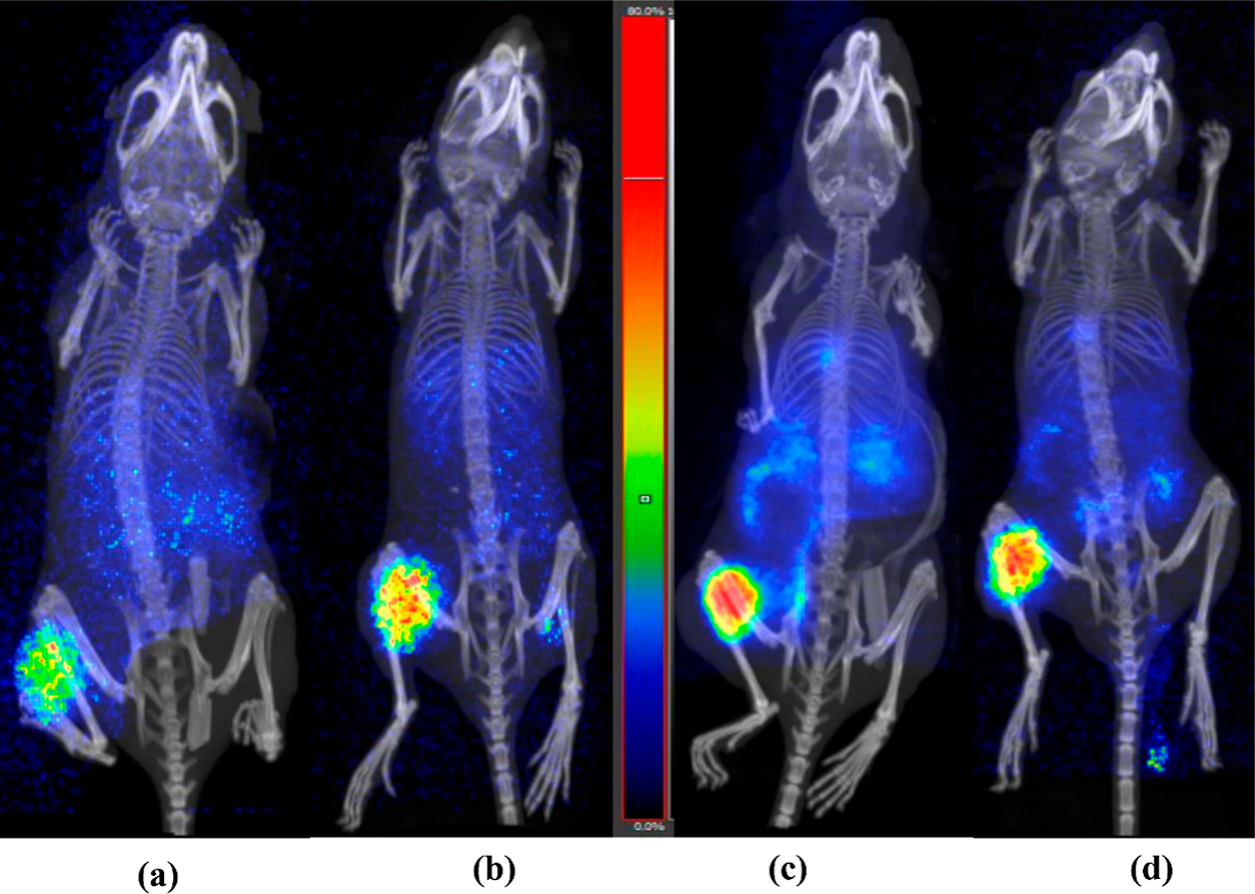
PET/CT images of: (a,b) [^68^Ga]Ga-NOTA-PEG2-[Sar^11^]RM26 (1 and 3 h pi, respectively) (c,d) [^68^Ga]Ga-NODAGA-PEG2-[Sar^11^]RM26 (1 and 3 h pi, respectively), in PC-3 xenograft bearing
